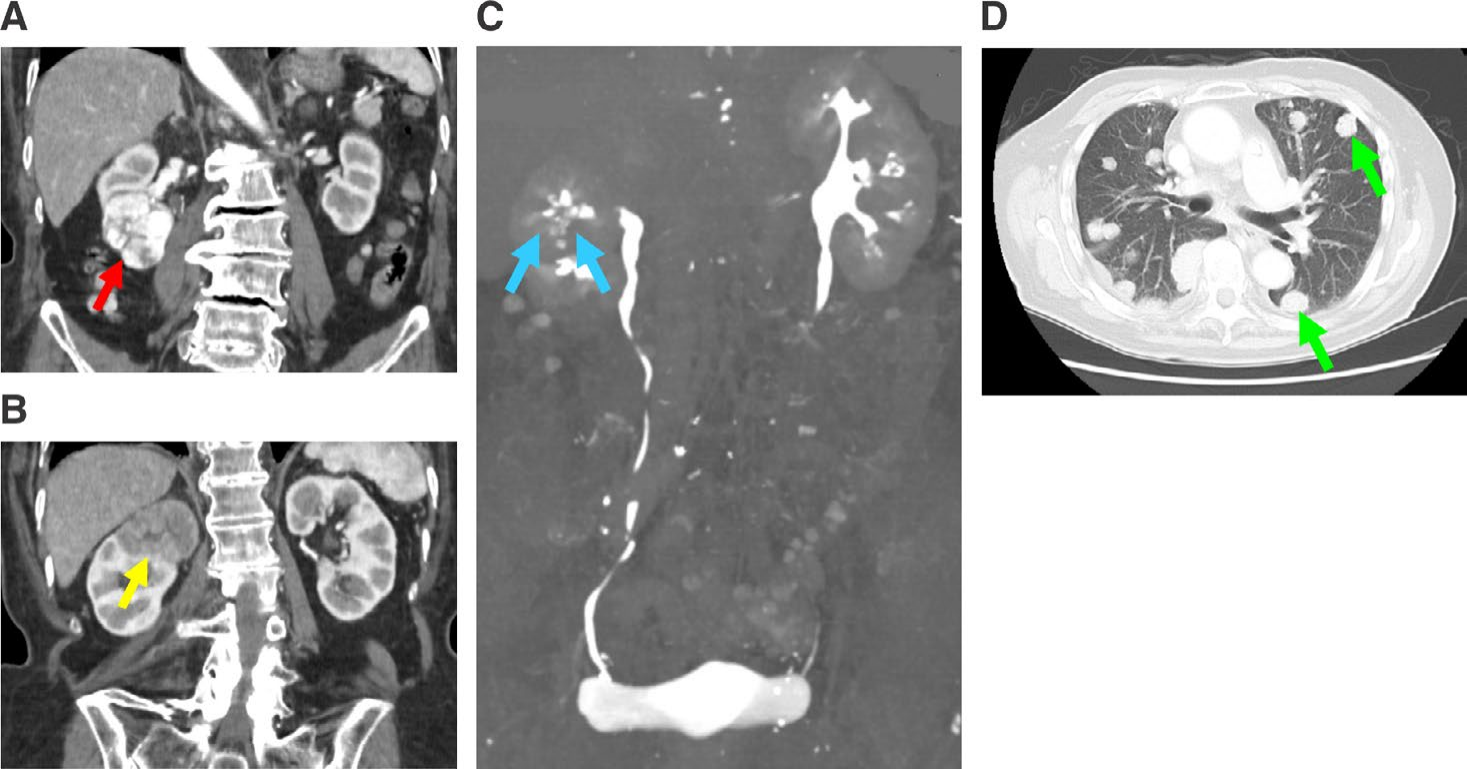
(A) Contrast‐enhanced abdominal CT reveals a strongly enhanced tumor measuring 45 × 41 × 39 mm at the lower pole of the right kidney during the arterial phase (red arrow). (B) Contrast‐enhanced abdominal CT reveals a poorly enhanced mass measuring 42 × 36 × 33 mm at the upper pole of the right kidney during the arterial phase (yellow arrow). (C) CT urography reveals a partial defect in the renal pelvis (blue arrow). (D) Plain chest CT reveals the presence of multiple lung tumors (green arrow).
